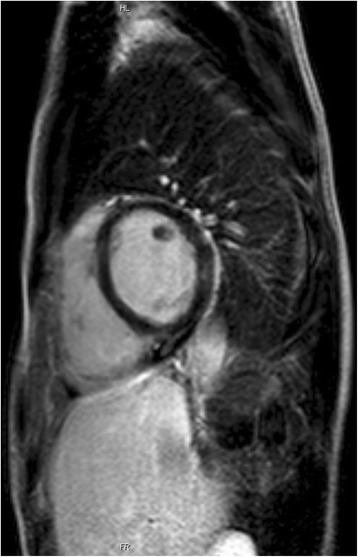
The Cardiac MRI shows a round mass adhering to the mitral valve
